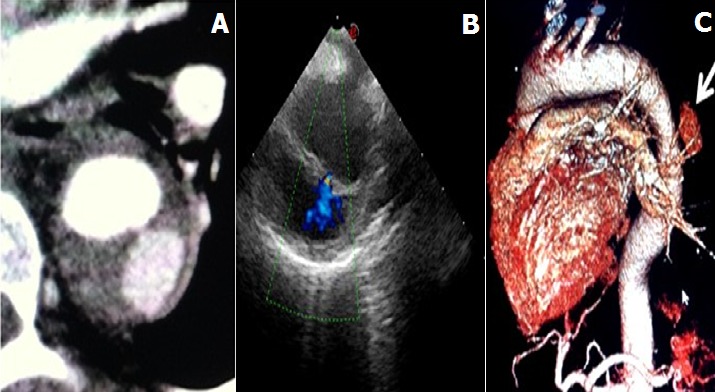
Intimal tear, with a reperfusion area in the hematoma. A) contrast enhanced CT scan; B) TEE; C) CT scan with reconstruction
